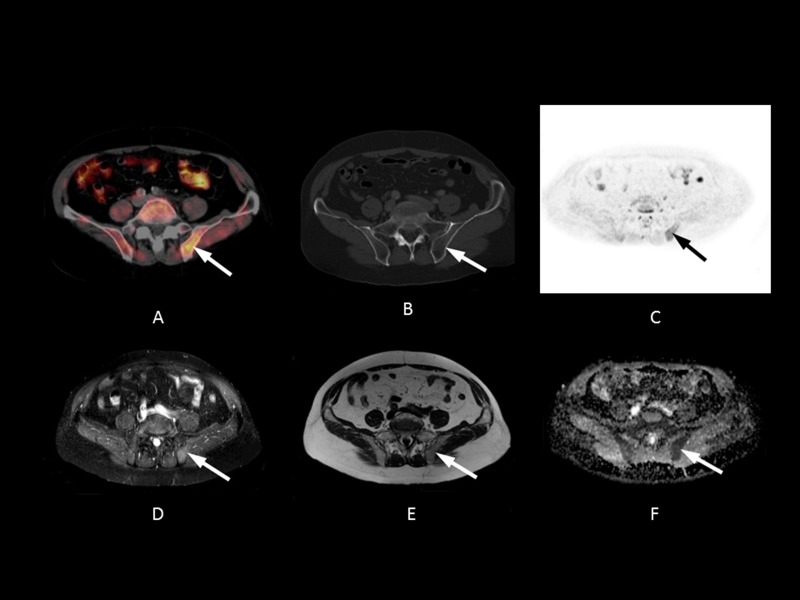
54-year-old patient with bone metastasis in the left pelvis nine years after radical prostatectomy (initial Gleason Score: 8) The PSA level at the time of imaging was 2.3 ng/mL. A fall in PSA level after pelvic irradiation confirmed a malignant lesion (1.0 ng/mL after radiation therapy). A. Axial PET/CT fusion image showing a focal 11C-choline uptake in the area of the posterior iliac bone (white arrow, SUV max 3.97). B. The lesion shows no substantial sclerosis (HU 708) and is hardly visible on CT. C. Axial 500 ms b-image and D. ADC map showing only a slight signal abnormality of the lesion in the left iliac bone (relative ADC lesion/normal bone: 1.02) (white and black arrows). E. The STIR image shows slight focal signal elevation (relative STIR SI lesion/normal bone: 0.90) and F. slight signal distortion can be seen on the T1w image (white arrow, relative SI lesion/normal bone: 1.05).
